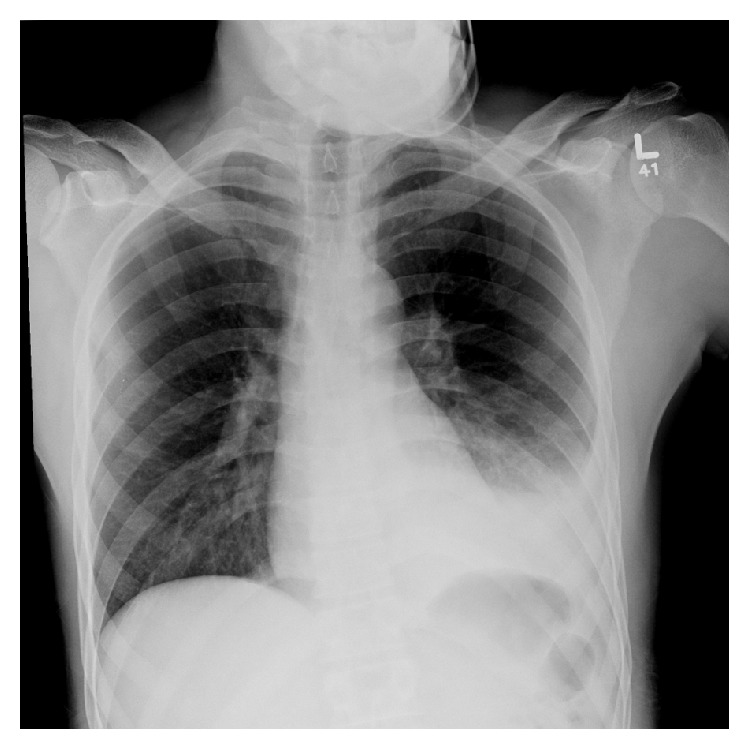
Chest X-ray imaging performed shortly after patient's arrival to emergency department displaying evidence of pleural fluid suspicious of hemothorax in the lower lobe of the left lung.
